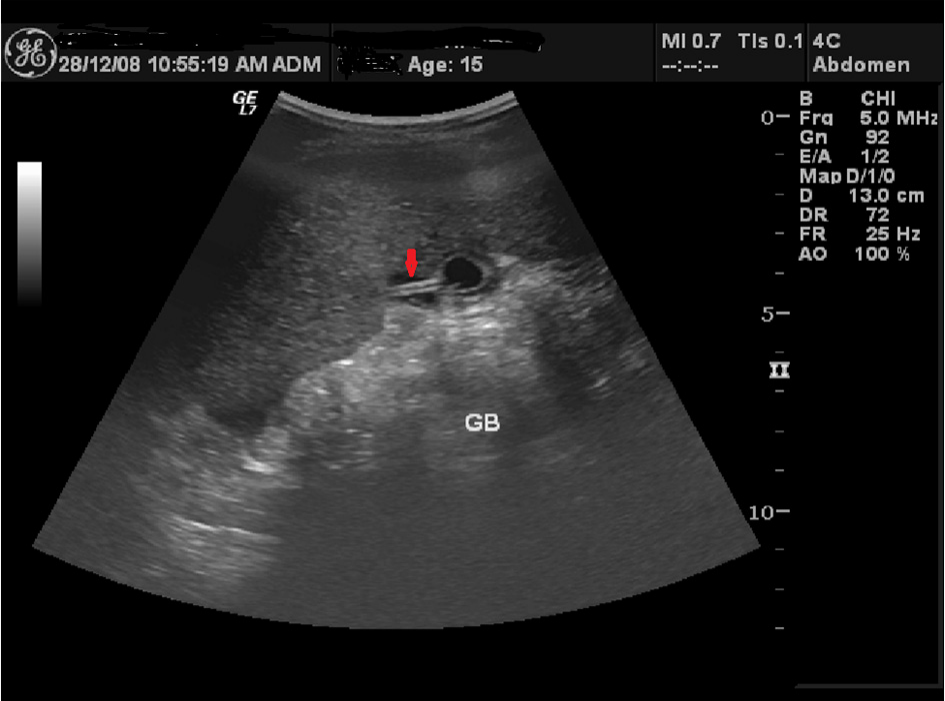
Ultrasound showing the Cholecystostomy tube inside collapsed GB (arrow).
